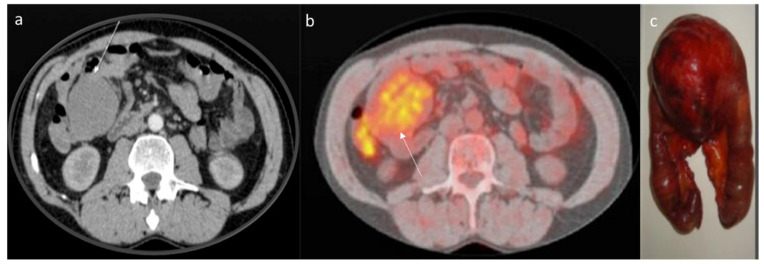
CT assessment (arrow) of mesenteric DT (a) in portal phase of contrast study. The lesion enhancement is mild to moderate. The 18-FDG (b) assessment (arrow) with moderate uptake. Surgical sample (c).
